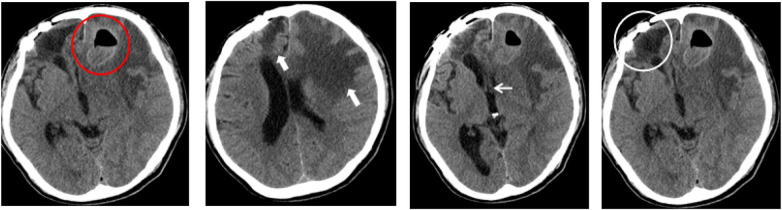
Pre-hospital CT: A unilocular cystic lesion is seen in the left frontal lobe, with gas inside the cyst and mixed-density cystic fluid (red circle); edema of the frontal lobe (thick arrow), marked midline shift (thin arrow); postoperative changes of the right frontal bone (white circle).
